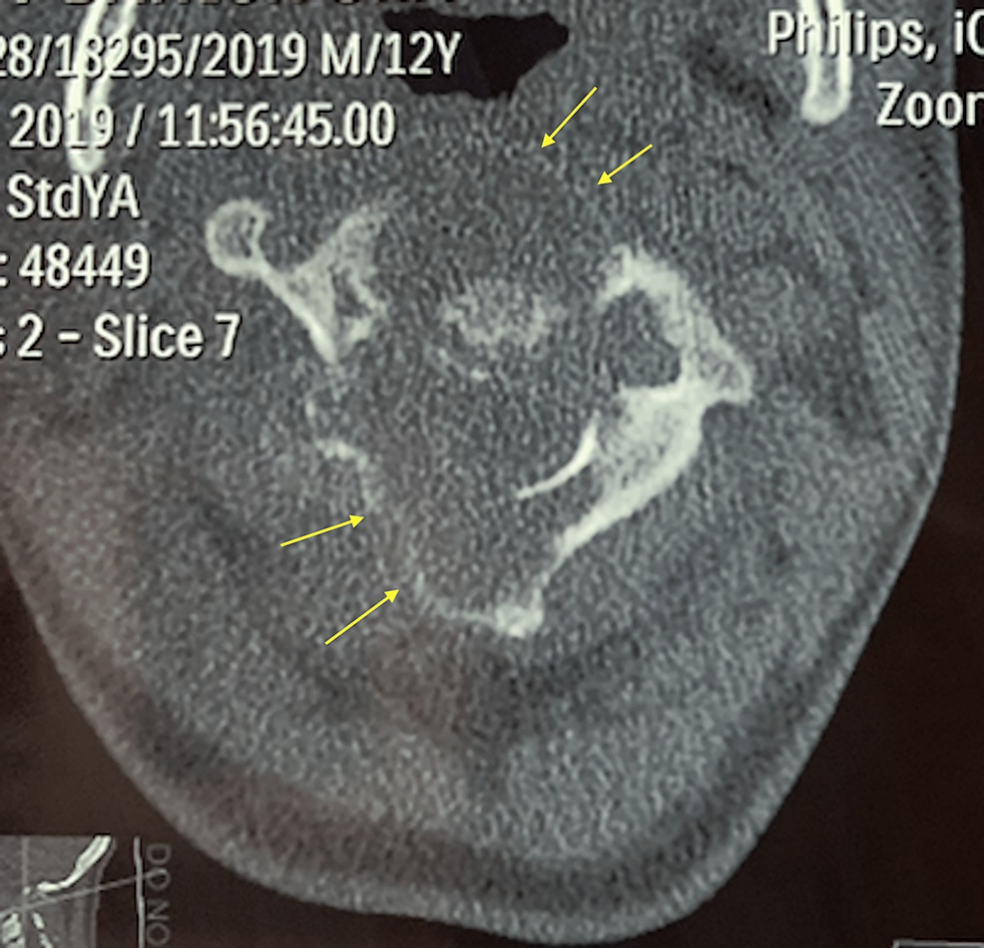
Axial CT of C2 vertebra Yellow arrows show the cortical borders which have been thinned out.
